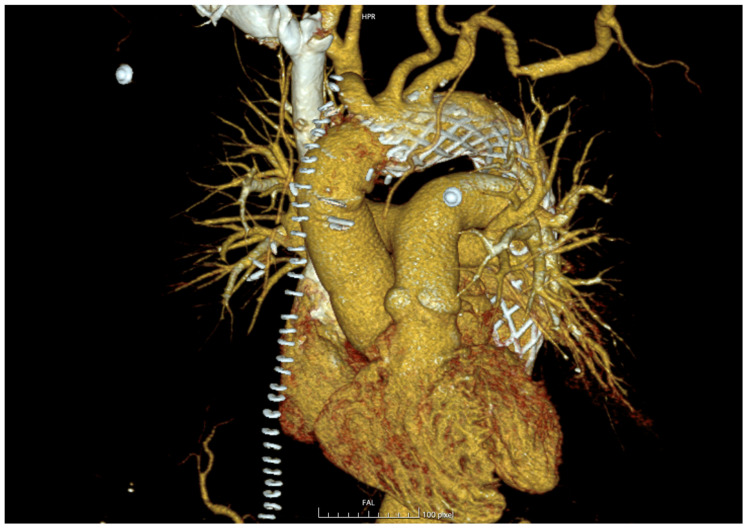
Three-dimensional postoperative CT reconstruction of the thoracic aorta showing an AMDS implanted at the aortic arch.
